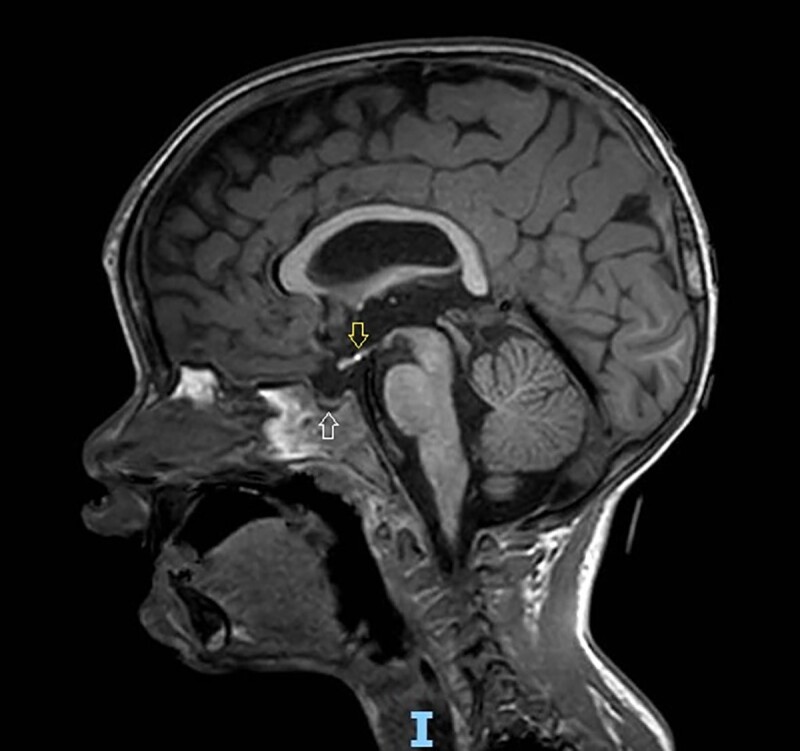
T1-weighted brain magnetic resonance imaging scan. Ectopic posterior pituitary at the level of median eminence (down arrow), thin pituitary stalk, and anterior pituitary hypoplasia (up arrow) in a 2-year boy with multiple pituitary hormone deficiency.
